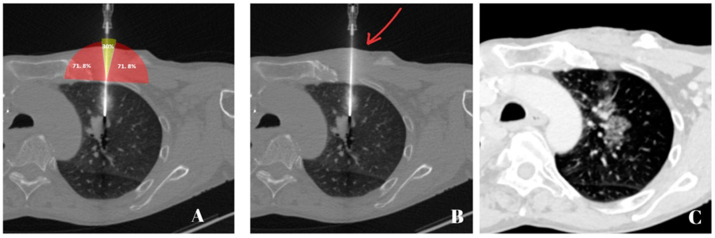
Different axial CT images of the same patient at 75 mA, 120 kV, and a 2 mm slice thickness with a 1 mm increment: (A) A safe zone scheme was placed on the needle before it entered the pleura. The yellow triangle depicts δ_min_ < 10° and the red triangles depict δ_min_ ≥ 10° with a significantly higher incidence of pneumothorax (71.8% vs. 30%); (B) The same image as presented in (A) but without the safe zone scheme. The red arrow points to the needle that has entered the lung at a 90° angle to the pleura, aiming for the presented pulmonary mass in the upper left lobe; (C) Post-intervention control scan showing no evidence of pneumothorax.
